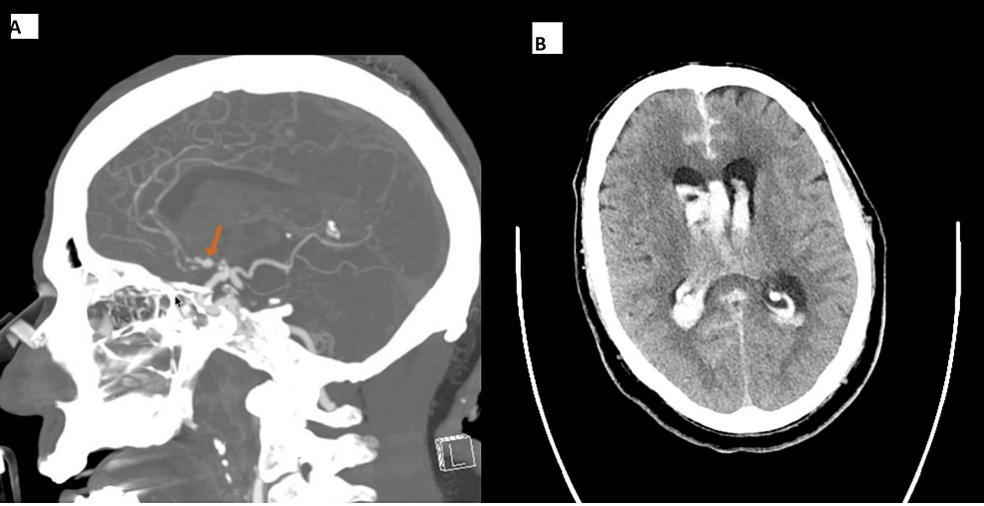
Computed Tomography Angiography of the Head and Neck (With Contrast) and Computed Tomography of the Head (Without Contrast) A: Arrow is pointing to anterior communicating artery aneurysm, demonstrating its close proximity to the hypothalamus B: Subarachnoid hemorrhage with intraventricular hemorrhages
